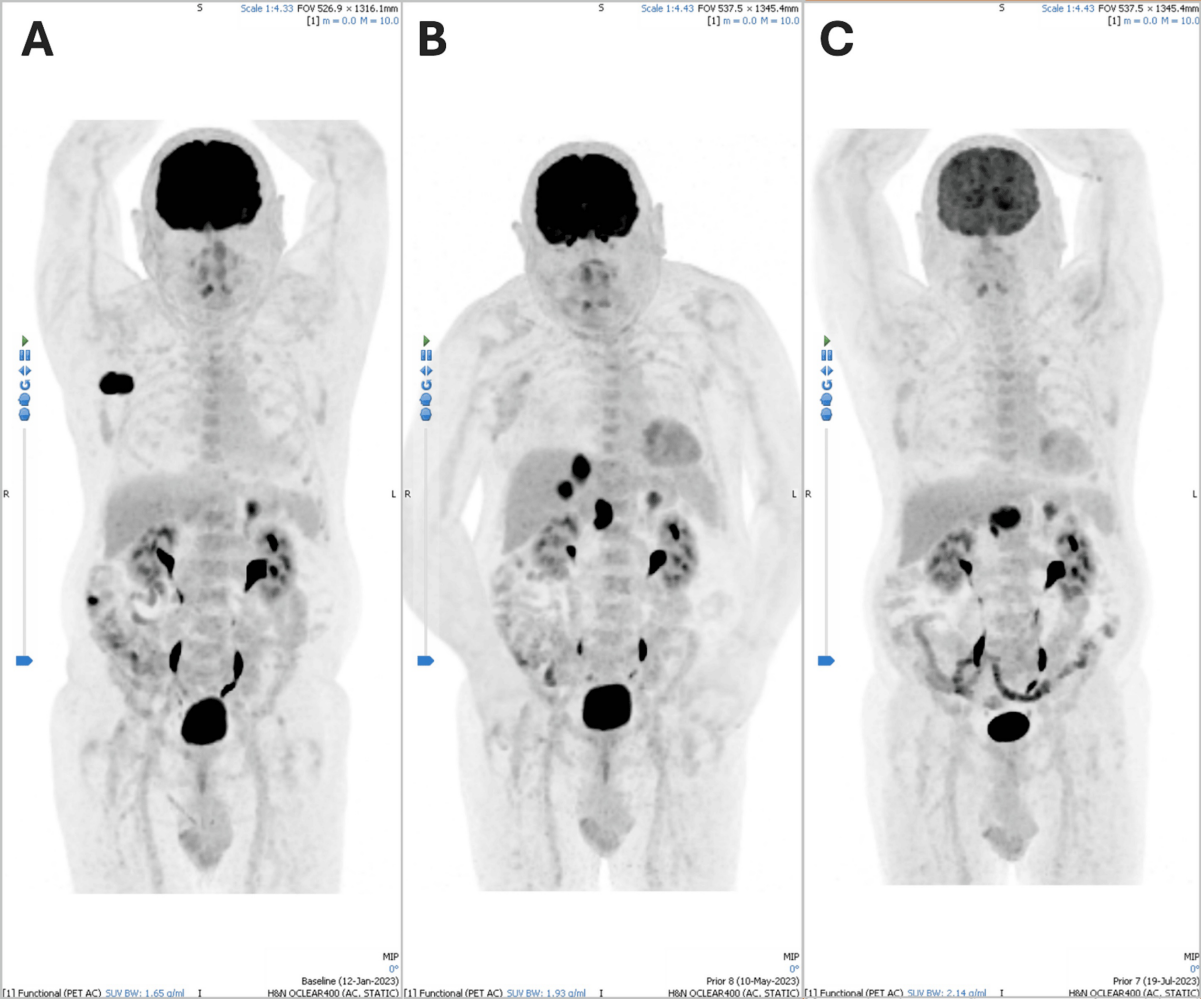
Positron emission tomography-computed tomography scan showing how the patient’s Merkel cell carcinoma was in remission at the time of his neurological symptoms. (A) The patient’s Merkel cell carcinoma (seen on his anterior right chest) at the time of his cancer diagnosis. (B) Excision of the chest lesion but metastases elsewhere, including the liver, shortly before initiating avelumab. (C) Image obtained on admission with his neurological symptoms, showing some remission of his Merkel cell carcinoma with improvement in his liver metastases after two doses of avelumab.
